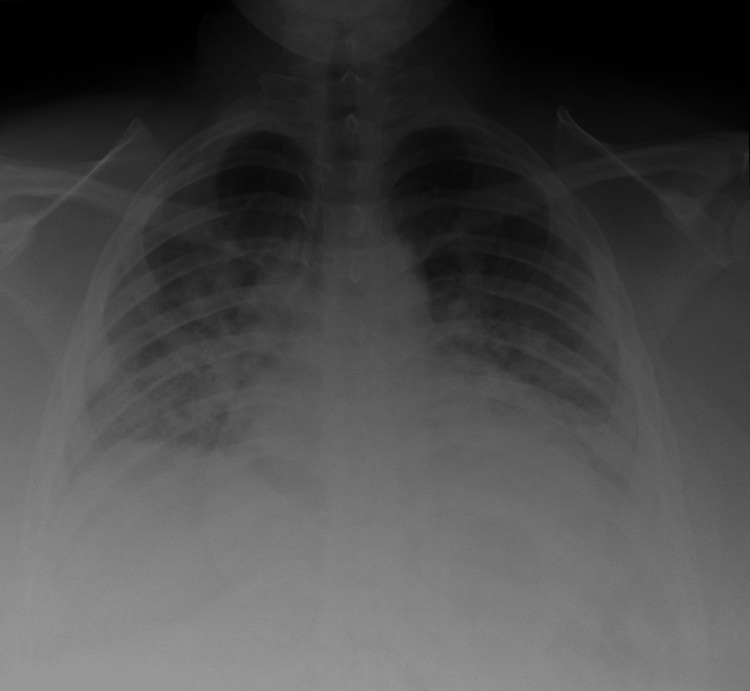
Chest radiograph two days later demonstrating marked improvement
